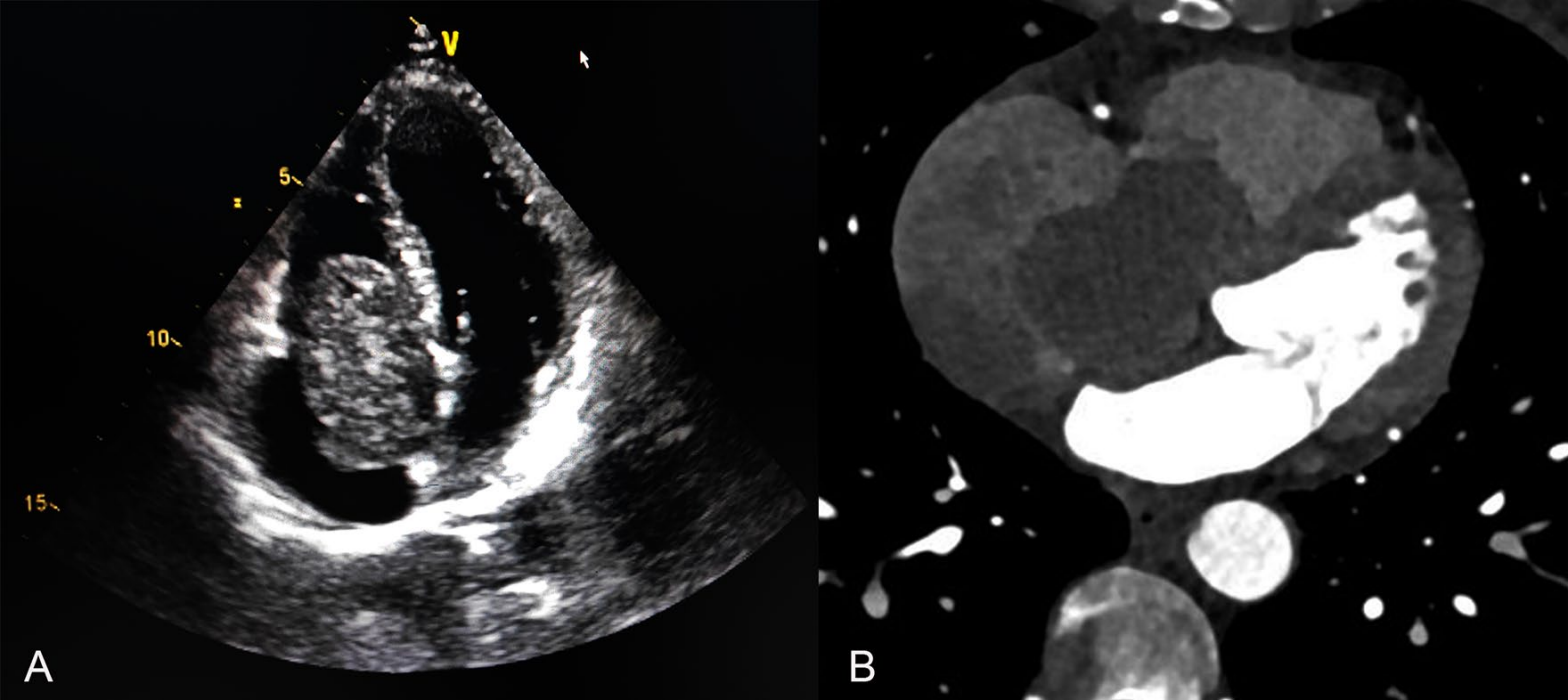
A Cardiac ultrasonography revealed an enormous tumor in the right atrium that is joined to the interatrial septum. B CT coronary angiography showed a mass extending from the right atrium through the interatrial septum to the right ventricle
